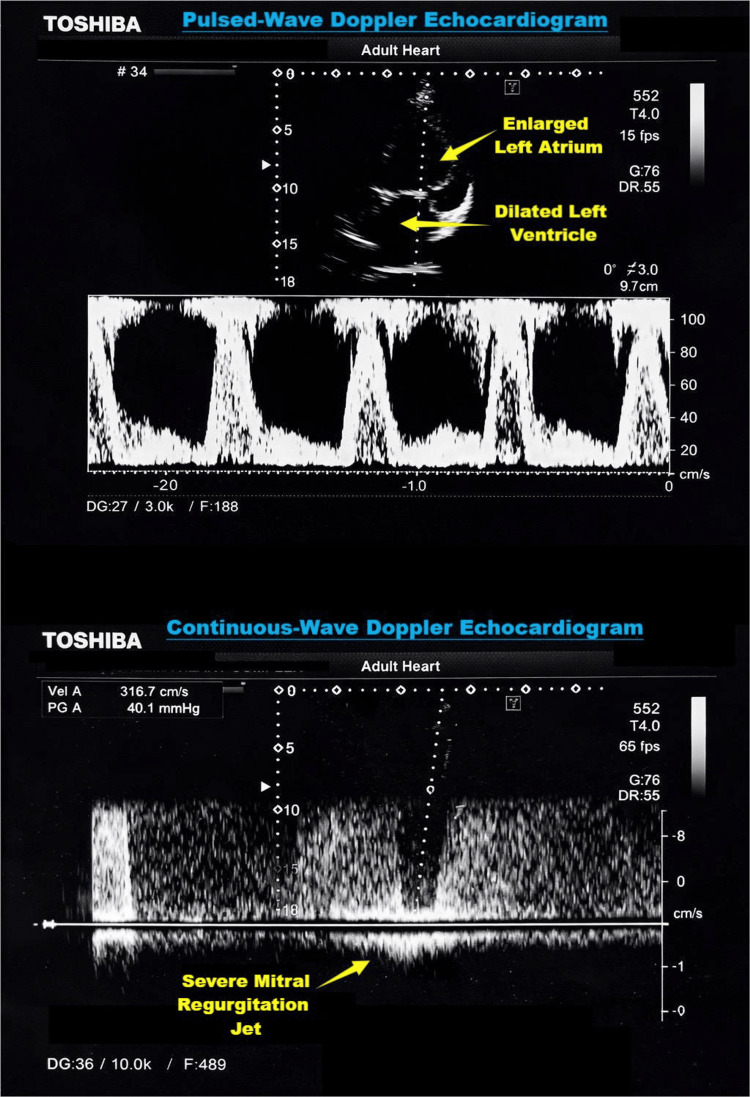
Transthoracic echocardiographic Doppler findings showing severe mitral regurgitation with left atrial enlargement and left ventricular dilatation The upper panel demonstrates pulsed-wave Doppler imaging, while the lower panel shows continuous-wave Doppler demonstrating a severe mitral regurgitant jet.
